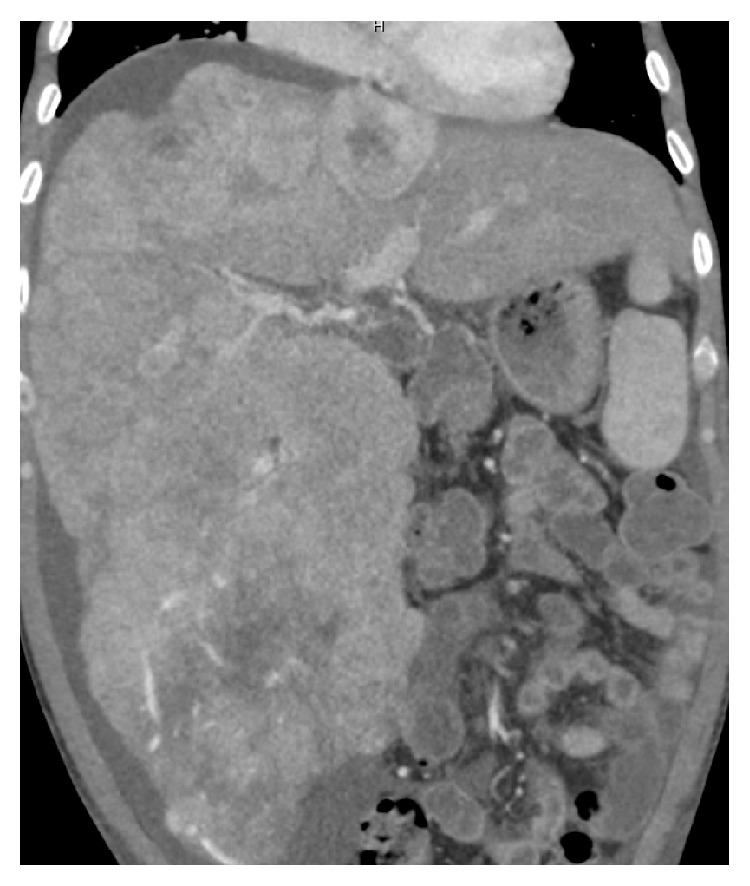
Multiphase abdominal computed tomography: Coronal view through the liver showing extensive multifocal HCC.
